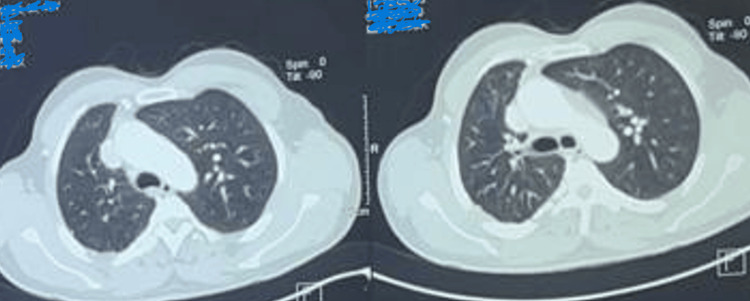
Chest CT parenchymal section: right lung hypoplasia and compensatory hyperinflation of the left lung
